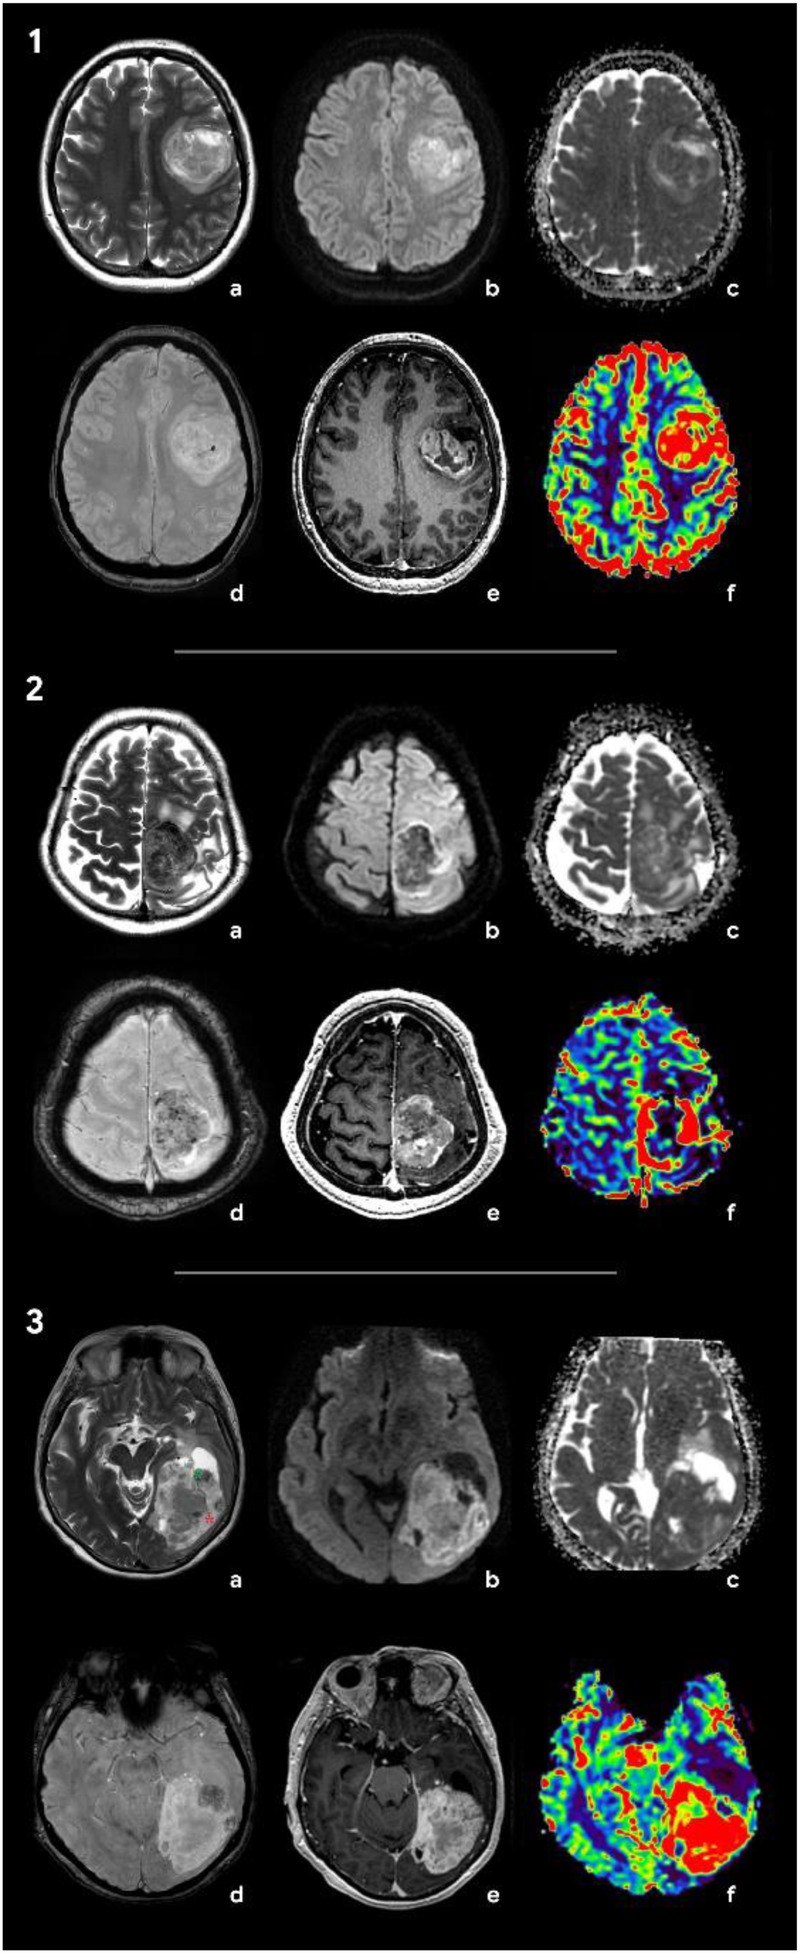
Radiologic features of the tumors included in differential diagnosis: glioblastoma (1), solitary metastasis (2), and anaplastic meningioma (3). (1) Brain MRI of a patient with glioblastoma: (a) Т2, (b) DWI, (c) ADC, (d) SWAN, (e) T1 + C, and (f) CBV. In the left frontal area, there is a large axial mass involving the cerebral cortex and white matter. The mass shows a ring-like contrast enhancement and contains necrotic areas. On SWAN, the vascular network and single foci of signal void are marked due to the presence of hemoglobin degradation products. On DWI and ADC maps, diffusion is restricted in the solid tumor component. The CBV map shows an increase in tumor blood flow. Т2 demonstrates a hyperintense signal of the tumor. (2) Brain MRI of a patient with brain metastasis of the lung carcinoma: (a) Т2, (b) DWI, (c) ADC, (d) SWAN, (e) T1 + C, and (f) CBV. There is an axial mass located in the left parasagittal region of the junction between the frontal and the parietal lobes. The mass shows a hypointense signal on T2WI and demonstrates vivid contrast enhancement. SWAN detects multiple artifacts caused by microhemorrhages. On DWI, there is a diffusion restriction alongside the tumor periphery. The CBV map shows a threefold increase in tumor blood volume relative to the white matter of the contralateral hemisphere. (3) Brain MRI of a patient with anaplastic meningioma: (a) Т2, (b) DWI, (c) ADC, (d) SWAN, (e) T1 + C, and (f) CBV. In the left occipital lobe, an axial mass with a wide dural base is identified. The tumor has a heterogeneous signal on T2WI with hypointense (green star) and hyperintense (red star) components. The tumor shows an intense and homogeneous enhancement and high blood volume values. SWAN depicts no magnetic susceptibility artifacts.
